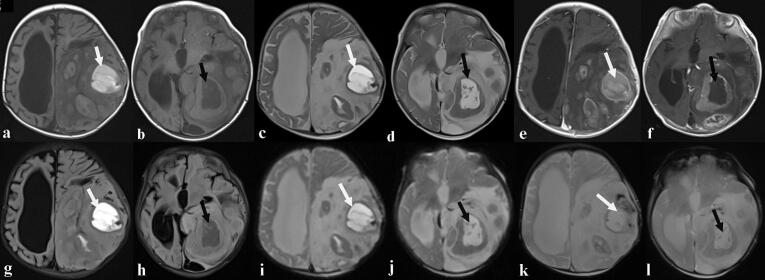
Axial MRI showing the large intra-axial tumor occupying almost the entire left hemisphere measuring approximately 11 × 9 cm in the axial plane and 11 cm in the sagittal plane. It had a heterogeneous signal on both T1 (a, b) and T2 weighted sequences (c, d), creating largely necrotic masses (b, d, f, h, l, black arrows) with hemorrhagic component (a, c, e, g, i, k, white arrows) and heterogeneous enhancement after gadolinium chelate injection (e, f). It is surrounded by perilesional edema responsible for a discrete midline shift to the right (g, h). The diffusion weighted sequence (i, j) showed a heterogeneous signal with hemorrhagic changes on the gradient echo sequence (k).
